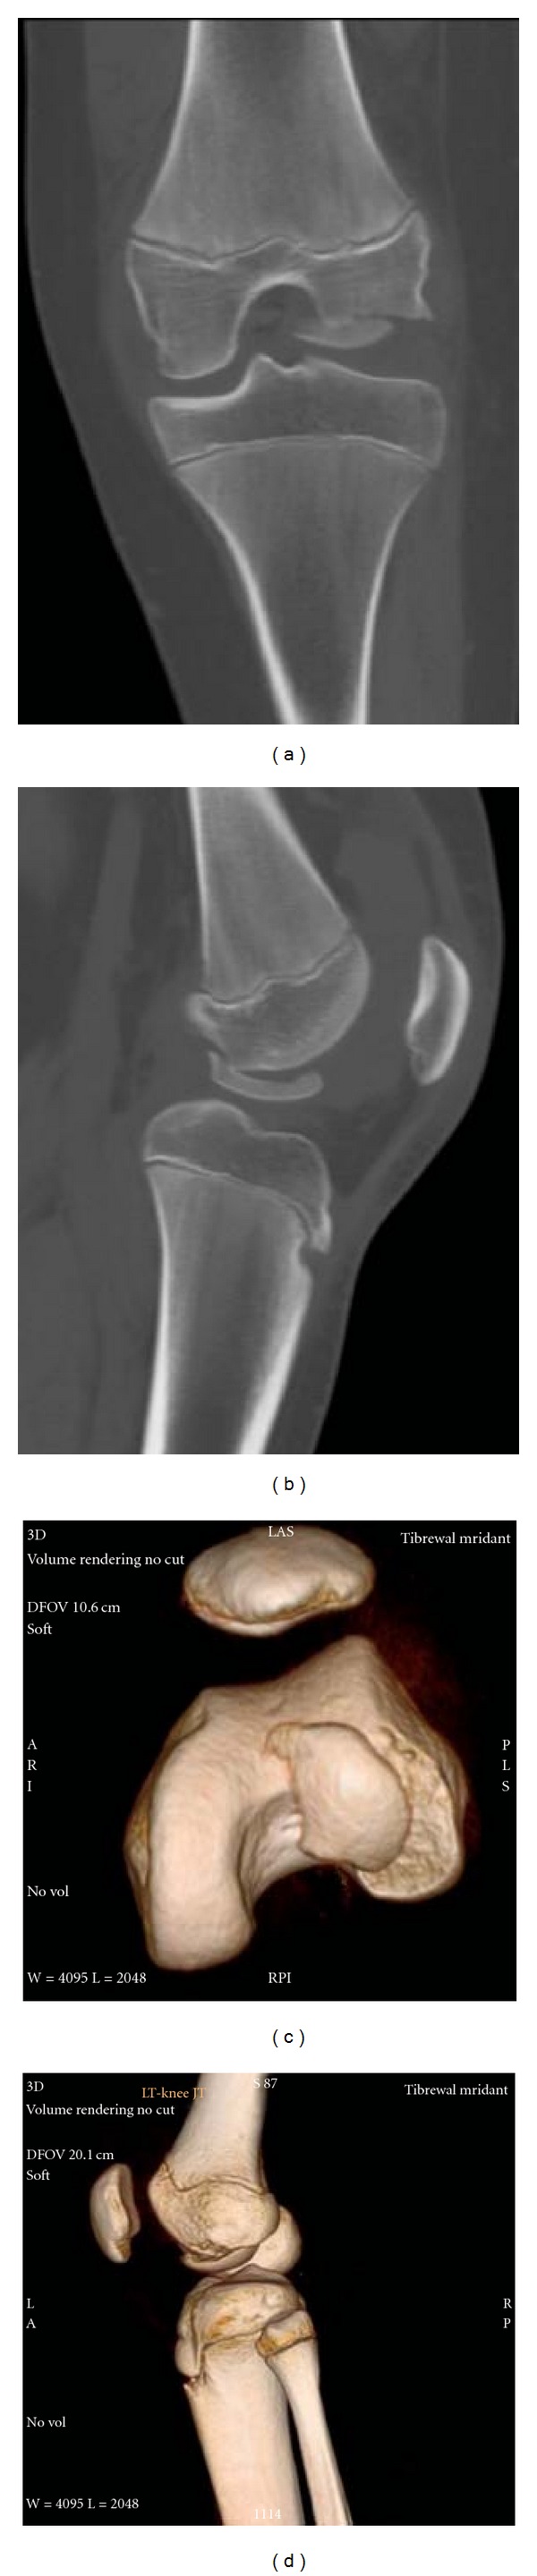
CT scan with 3D reconstruction.
